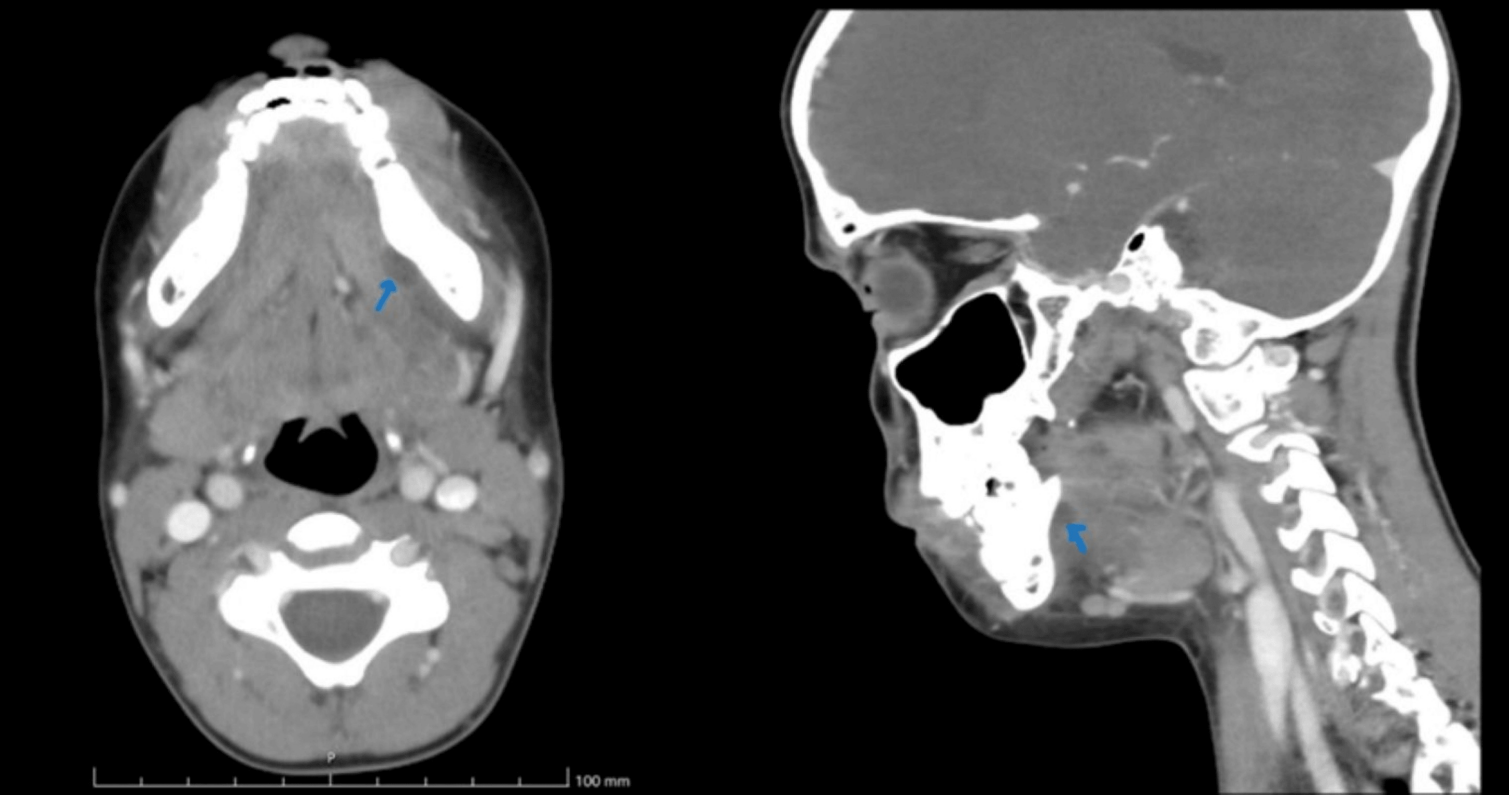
CT scan (Day 3) showing enlargement of the left submandibular gland with hypodensity in the left sublingual space near tooth 36 (blue arrows). Extensive crown erosion and periradicular lytic lesions are also observed, consistent with an odontogenic abscess and Ludwig's angina.
